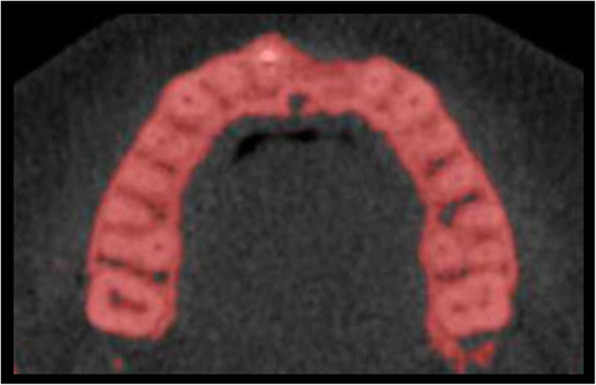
CBCT scan (axial view) of lower jaw at time point T0 before onlay graft
